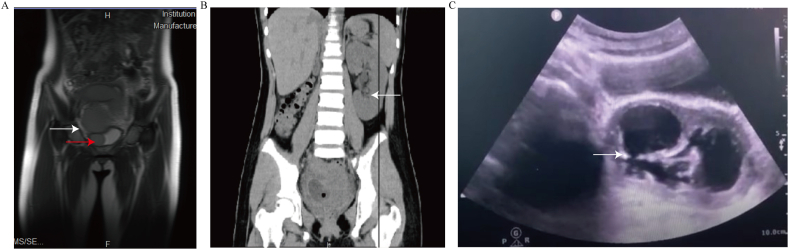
MRI, CT, and transabdominal ultrasound manifestations of the patient. (A) The right ureteral remnant partly forms a fistula tube communicating with the vagina and bladder (white arrow). The enlarged part of the ureteral remnant merged with the vagina (red arrow). (B) The right kidney agenesis and left kidney enlarged (white arrow). (C) Two vaginal oblique septa. The inner part communicates with the outer one through a valve-like structure (white arrow).
